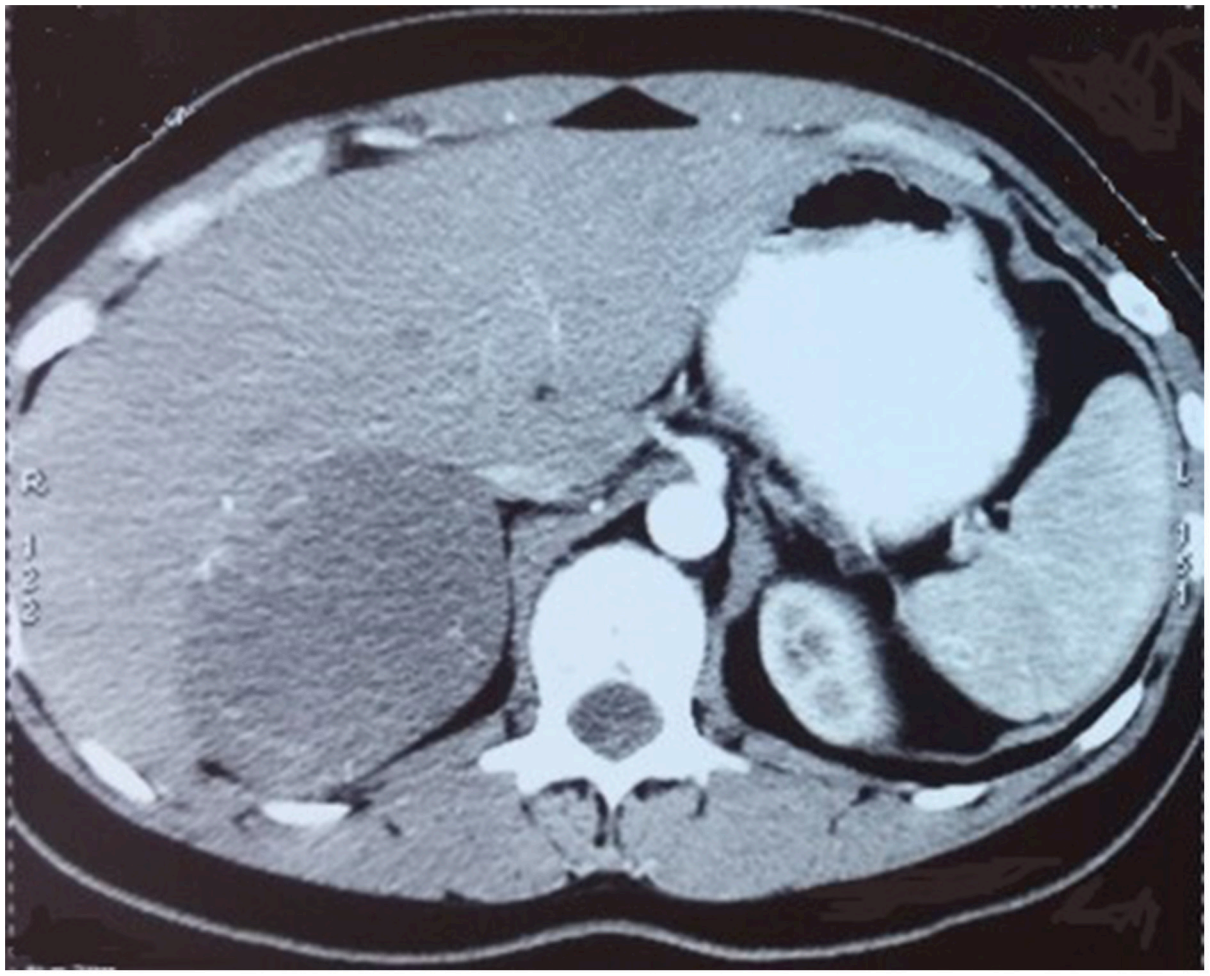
CT scan showed a round mass between liver and right kidney with heterogeneous enhancement.
